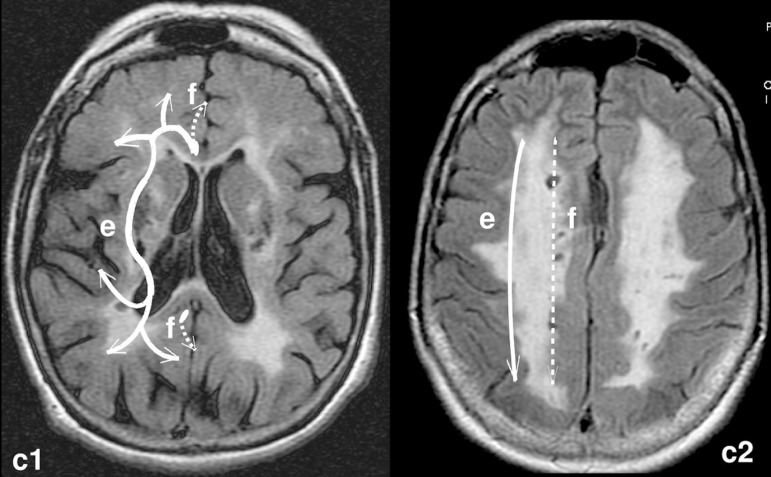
RM-FLAIR. Axial sections of the brain of a case with extensive
